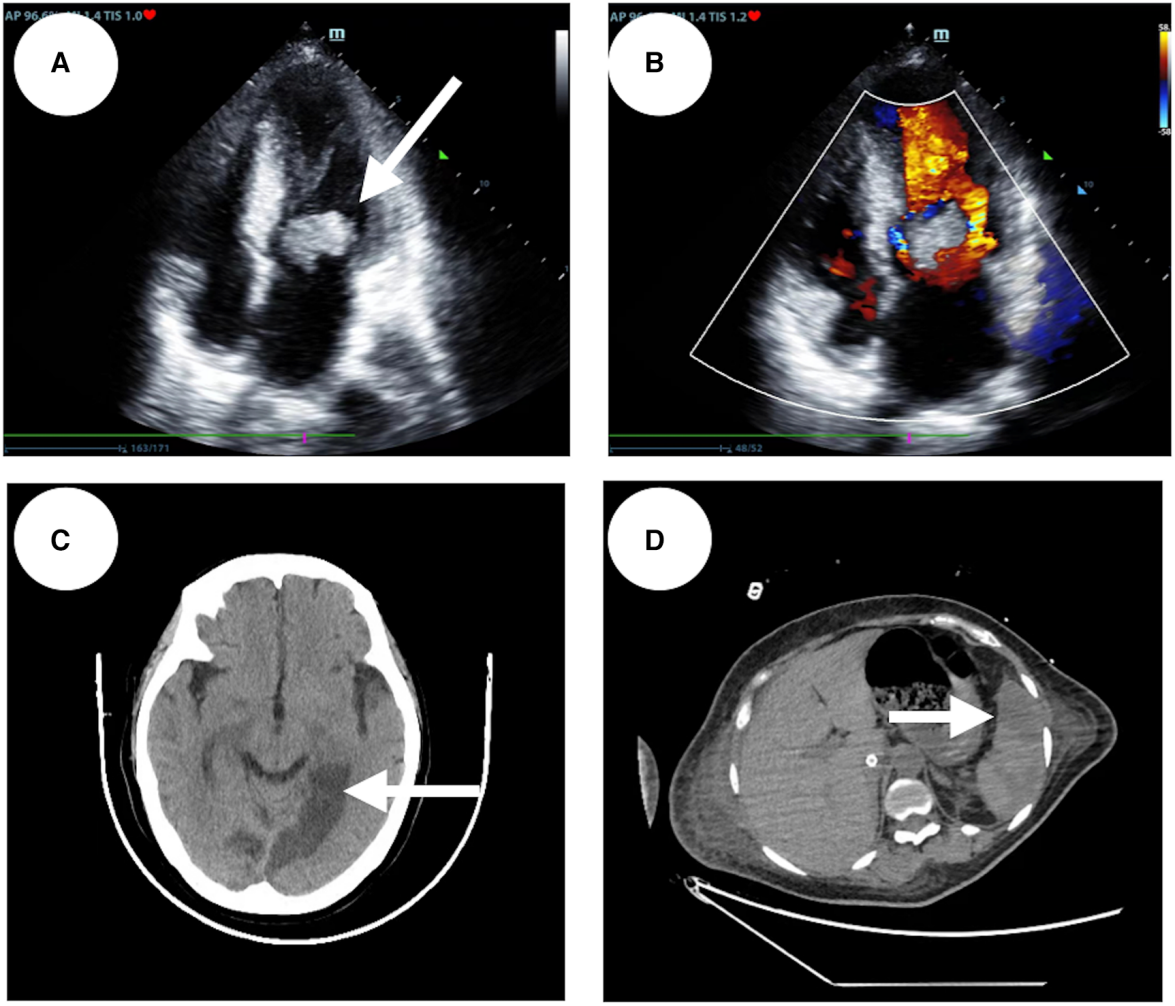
Patient's emergency echocardiography, abnormal CT scans of the patient's head and abdomen. (A) The patient's apical four-chamber view of echocardiography. (B) An irregular isoechoic mass of approximately 45 × 27 mm in size was attached to the anterior mitral valve (indicated by the white arrow). (C) On the left side of the anterior horn of the lateral ventricle, the left thalamus, the left parietal-occipital lobe, and the left side of the splenium of the corpus callosum, a patchy low density shadow with clear boundaries is visible (indicated by the white arrows). (D) Patchy low-density shadow (indicated by the white arrows) on the spleen.
